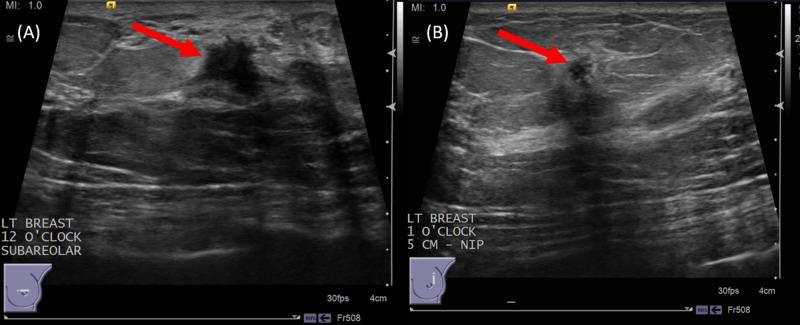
Left breast diagnostic ultrasound (A) Irregular mass measuring 10 x 6 x 8 mm on ultrasound at subareolar 12 o'clock region (red arrow); findings represent a suspicious abnormality, Breast Imaging-Reporting and Data System (BI-RADS) category 4C. (B) Irregular mass measuring 5 x 4 x 5 mm on ultrasound at 1 o'clock region, 5 cm from nipple (red arrow); findings represent a suspicious abnormality, BI-RADS category 4B.
